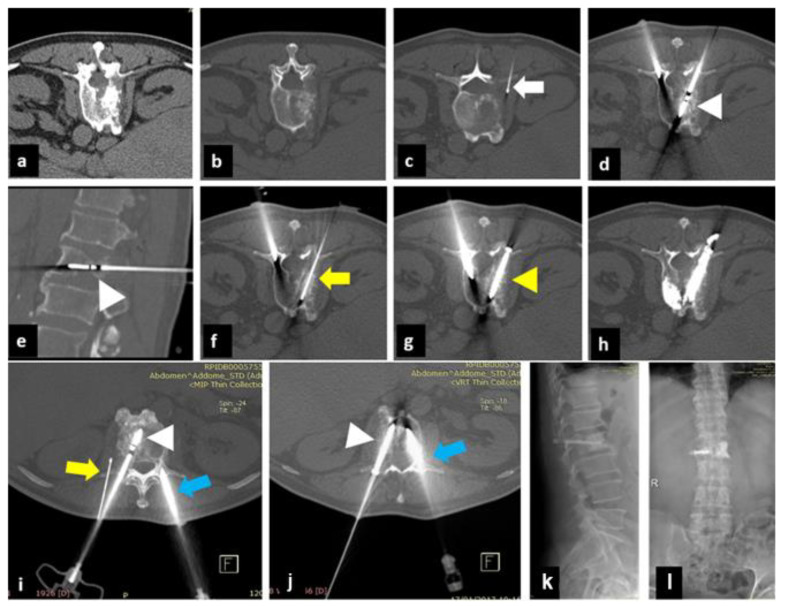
A 65-year-old man with a history of small cell lung carcinoma. (a,b): Axial CT shows extensive osteolytic metastasis of the L2 vertebral body involving the right pedicle. (c): Axial CT shows thermocouple placement in the left root between L2 and L3 (white arrow). (d,e): Axial and sagittal CT, MW antenna placement in the tumor for thermal ablation. Contralateral initial placement of the VTP needle (white arrow). (f): Axial CT shows k-wire placement for subsequent coaxial screw insertion (yellow arrow). (g): Axial CT shows placement of the left screw (yellow arrow) and contralateral advancement of the vertebroplasty cannula (blue arrow). (h): Axial CT shows cementing of the screw (yellow arrowhead) and contralateral vertebroplasty (blue arrow). (i,j): Axial CT with Maximum Intensity Projection (MIP) reconstruction showing the MW antenna (white arrow) and thermocouple (yellow arrow) positioned and the vertebroplasty cannula in contralateral extrapedicular location (blue arrow). (k,l): Postoperative 3-month radiograph of the thoracolumbar spine in antero-posterior and latero-lateral projection after microwave ablation of the L2 vertebra combined with pedicle screw fixation followed by vertebroplasty (MASFVA).
